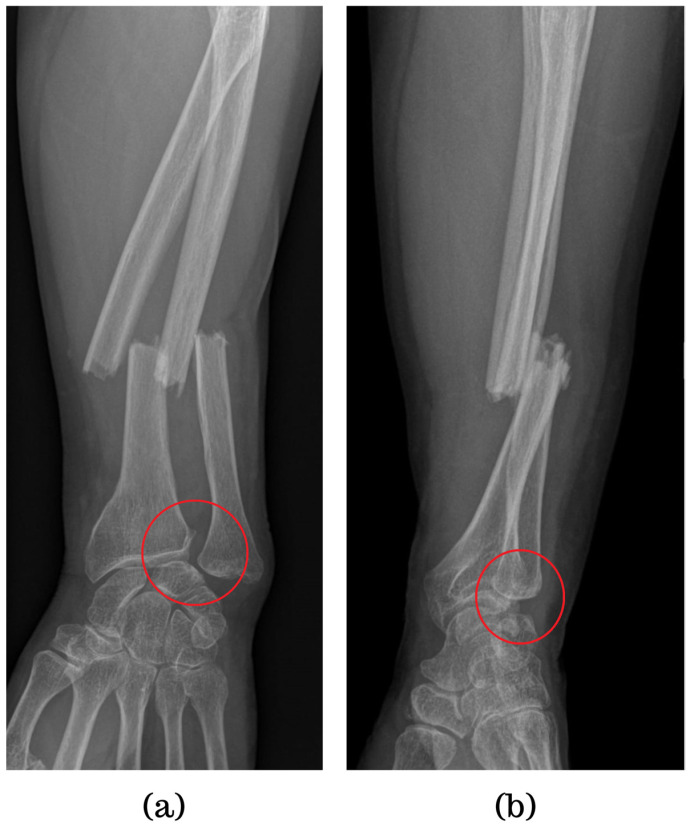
Forearm fracture with radiographic evidence indicating distal radioulnar joint (DRUJ) injury: (a) antero-posterior view (increased radioulnar space); (b) lateral view (dorsal dislocation of the ulnar head).
